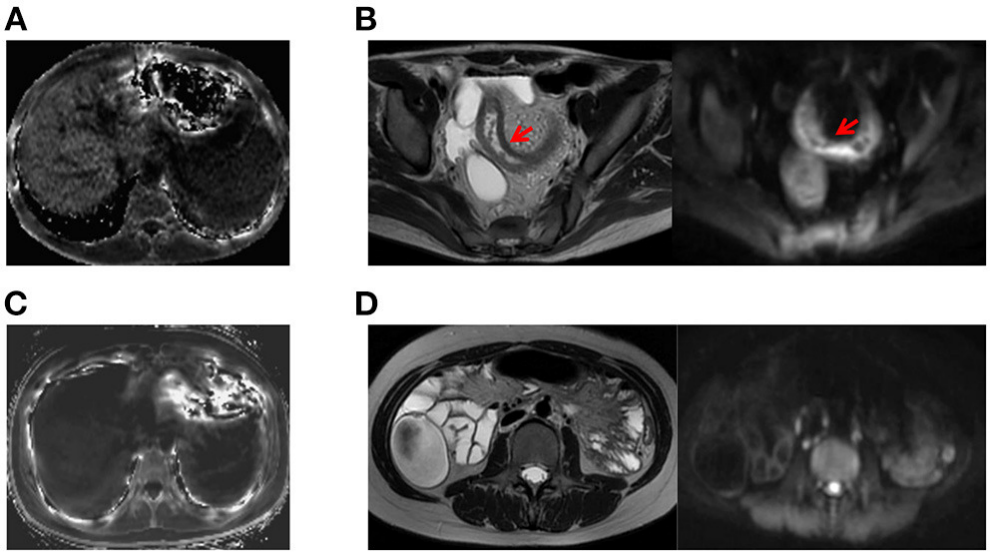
Examples of comparative MRE images obtained from patients with active CD and inactive CD. (A) A patient with active CD who showed higher liver signal intensity on the R2* map (R2* = 93.074; CDAI value, 233). (B) Corresponding T2-weighted and diffusion-weighted images of a patient with active CD showing inflammation of the bowel wall, including thickening of the wall of the ileum with an increased signal, rough mucosal surface, and a small amount of pelvic cavity effusion. (C) MRE image of a patient with inactive CD on the R2* map (R2* = 14.12, CDAI value, 73). (D) Corresponding T2-weighted and diffusion-weighted images for a patient with inactive CD. CD, Crohn's disease; CDAI, Crohn's Disease Activity Index; MRE, magnetic resonance enterography.
